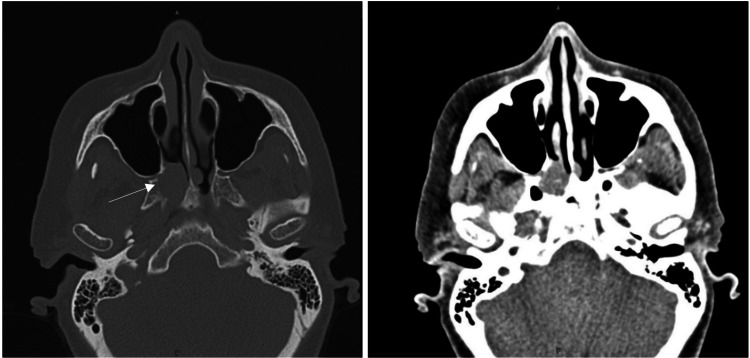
Non-contrast CT of the paranasal sinuses. Soft tissue mass centered at the right posterior nasal aperture (choana) with associated osseous scalloping and erosion of the medial margin of the right medial pterygoid plate.
